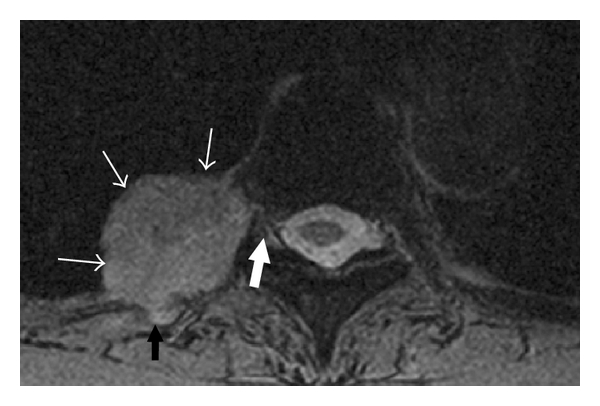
Axial T2-weighted MRI in a 66-year-old male through the upper right hemithorax demonstrates a soft tissue mass (white arrows) in the right pleural space. The lesion is observed to invade the chest wall and adjacent rib (black arrow). Note the proximity of the mass to the intervertebral foramen and spinal canal (thick white arrow).
